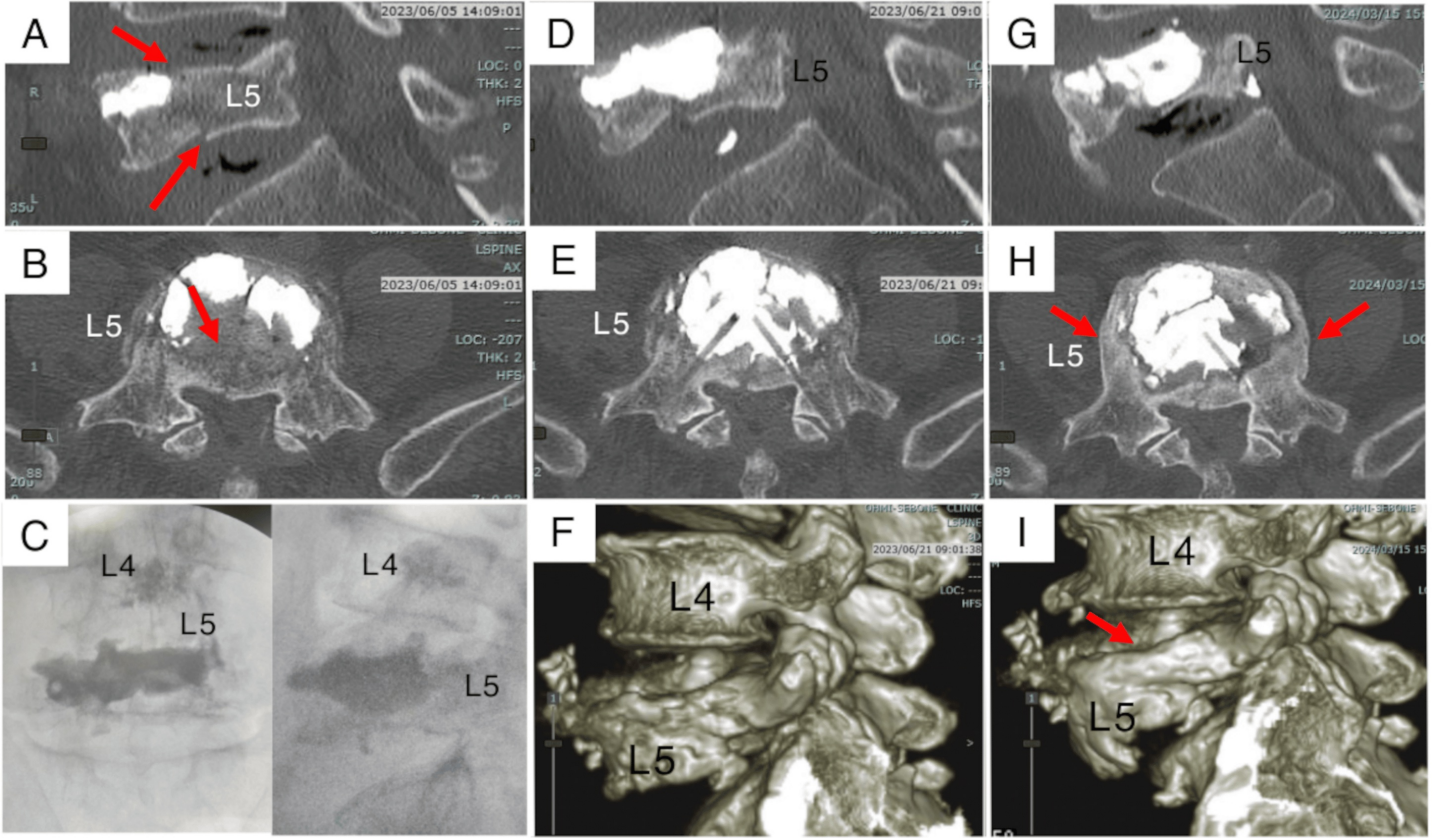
Preoperative and postoperative images of the revision balloon kyphoplasty (A, B) A new fracture (red arrows) is evident dorsal to the cement from the initial balloon kyphoplasty. (C) An additional 9 mL of cement was injected during the revision balloon kyphoplasty. (D, E) The additional cement covers the secondary fracture. (G, H) Ten months post-revision, osteogenesis and remodeling (red arrows) are observed in the lateral wall of the L5 vertebral body. (F, I) Three-dimensional reconstructed computed tomography images demonstrate bony fusion.
